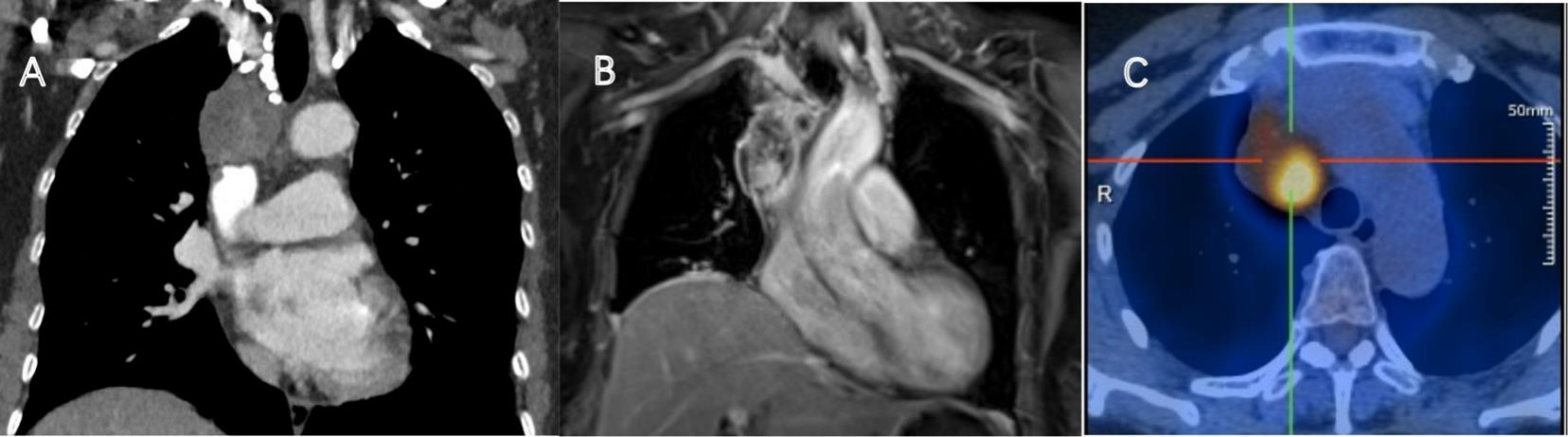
Multimodal images of a 39-year-old woman with a right anterior superior mediastinal mass. Coronal-enhanced CT revealed tumor invasion at the brachiocephalic vein-superior vena cava junction (A), MRI showed moderately heterogeneous contrast enhancement (B), and PET-CT detected hypermetabolic activity in the upper mediastinum (SUVmax = 9.2) (C).
